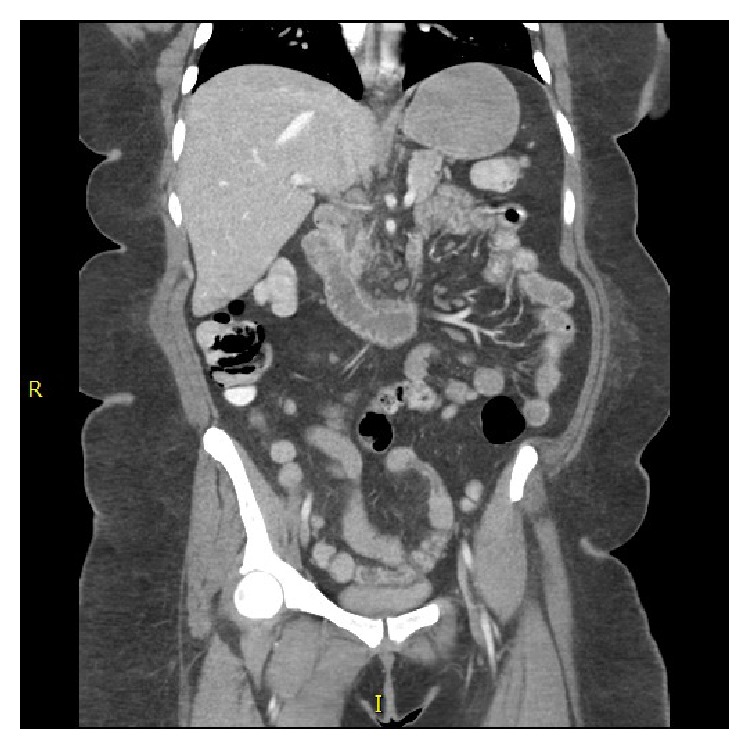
Showing Pneumatosis Intestinalis in right lower quadrant in a coronal cut of CT imaging.
